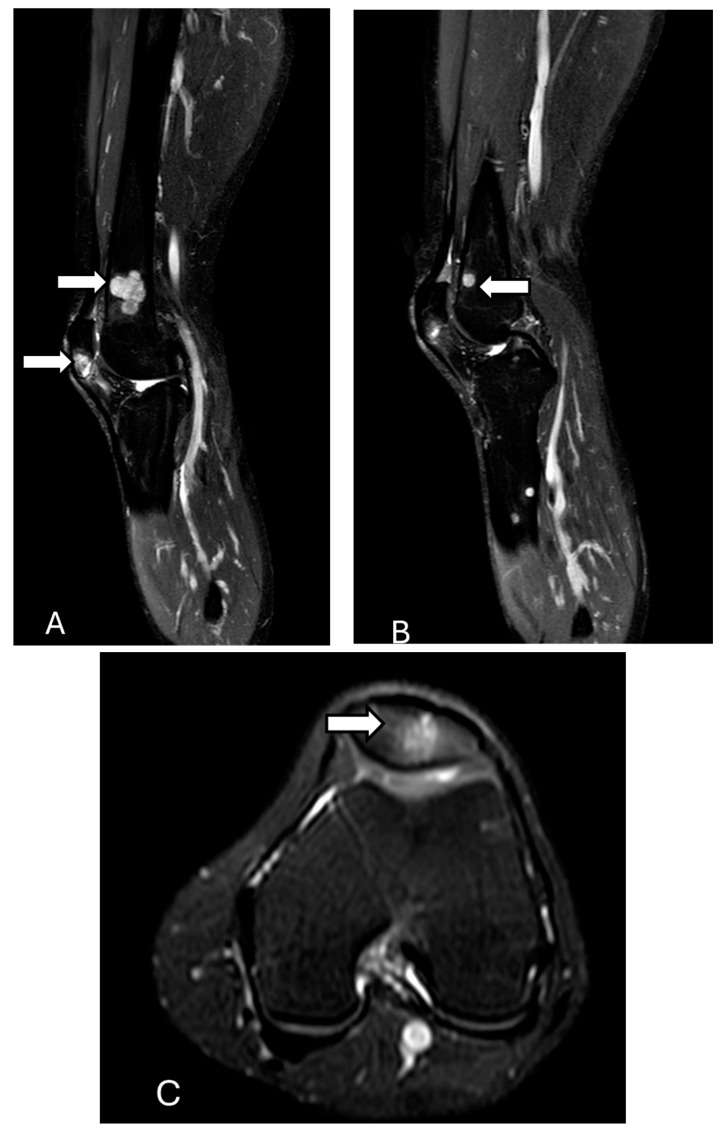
Epitheloid hemangioendothelioma. Sagittal (A,B) and axial (C) T1-weighted fat-suppressed post-contrast images show multiple foci of enhancement (arrows) throughout the distal femur, patella, and proximal tibia and fibula. These lesions are both medullary and cortically based with well-defined borders. Within the inferior pole of the patella, a similar lesion is observed. There is associated cortical thinning without evidence of cortical breakthrough. Findings are consistent with multiple metastasis with origin of epitheloid hemangioendothelioma. The images were obtained from the University of Washington Medical Imaging database with the required permissions.
